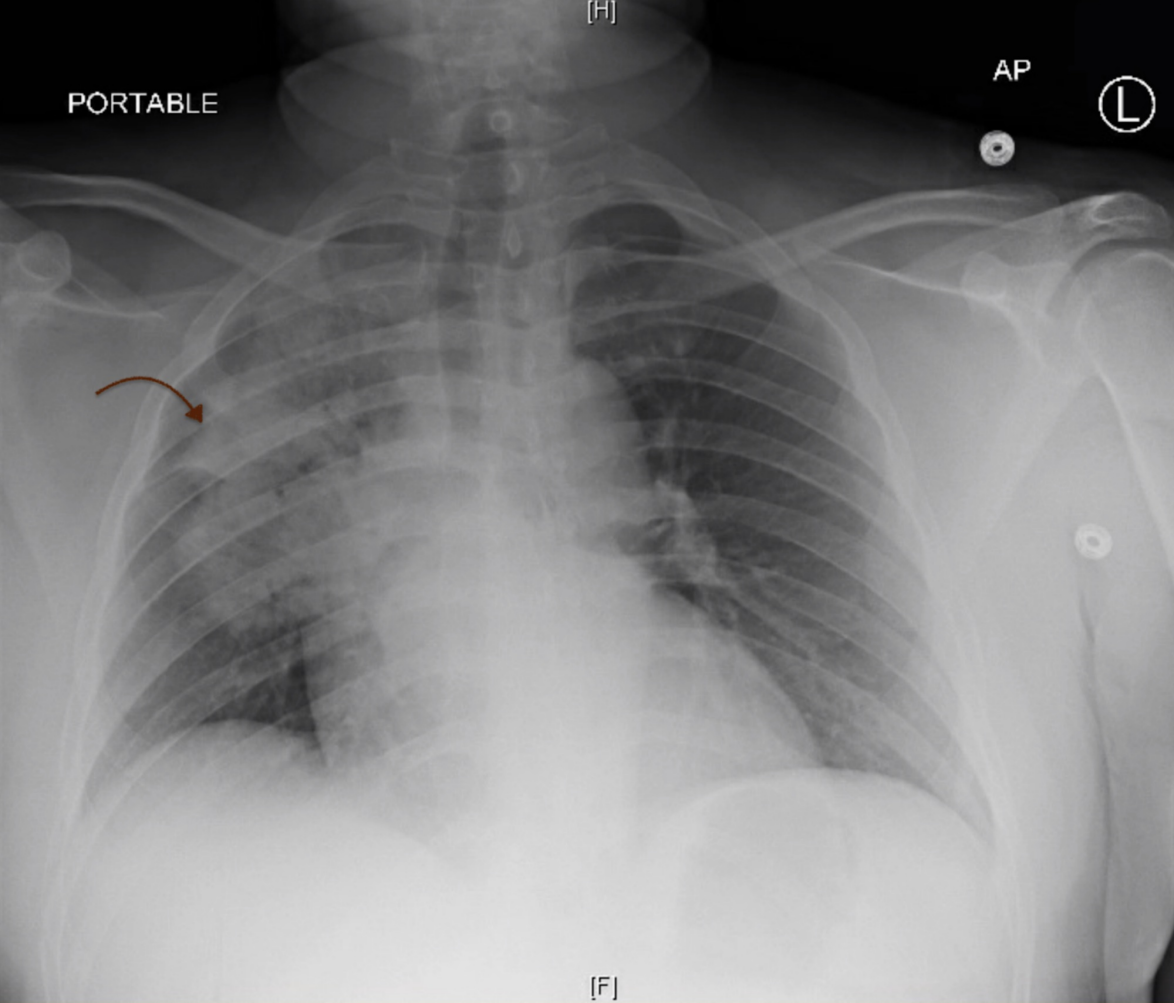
Chest X-Ray Consolidation found in the right upper and middle lobes with the presence of air bronchograms. H, head; F, foot, AP, anterior/posterior view.
